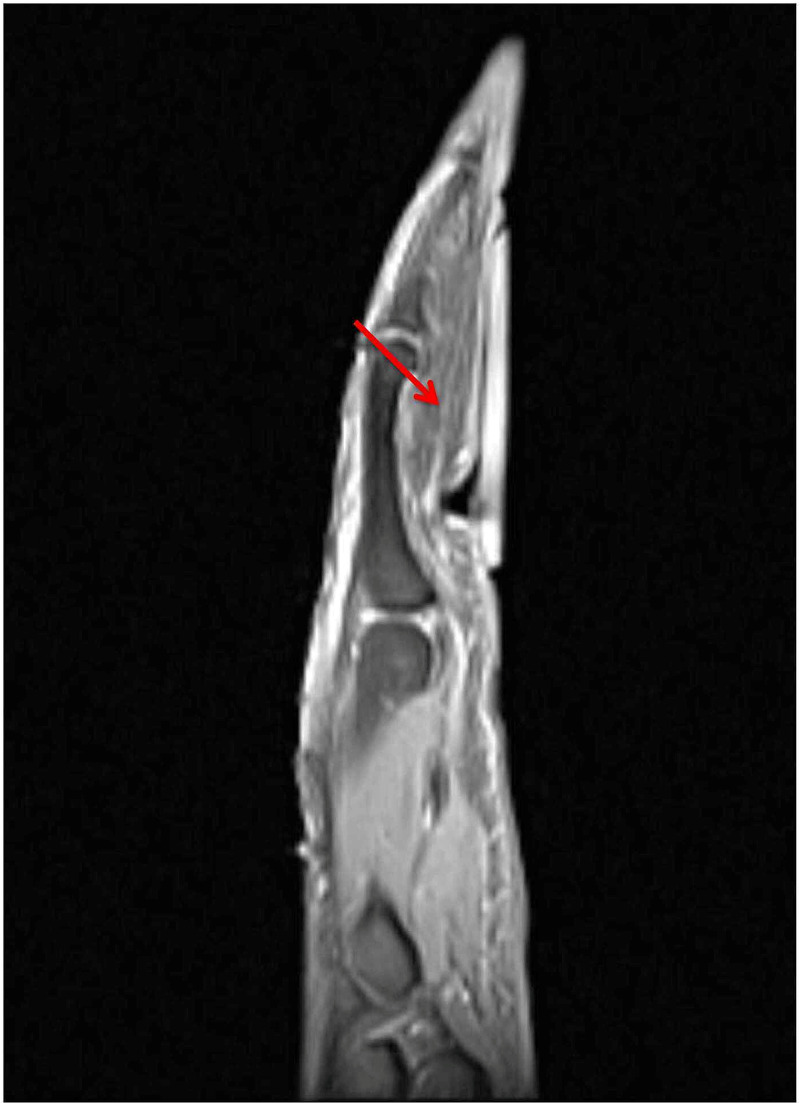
Sagittal MRI imaging of the right hand without gadolinium contrast enhancement showed intraneural lipoma extending along volar right ring finger MRI: magnetic resonance imaging
